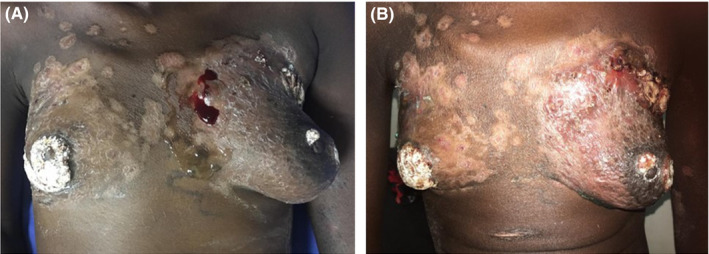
A and B demonstrated hypopigmentation of skin in nonuniform pattern with dried whitish powder covering both nipples. The bleed in the left upper inner quadrant during echocardiography
